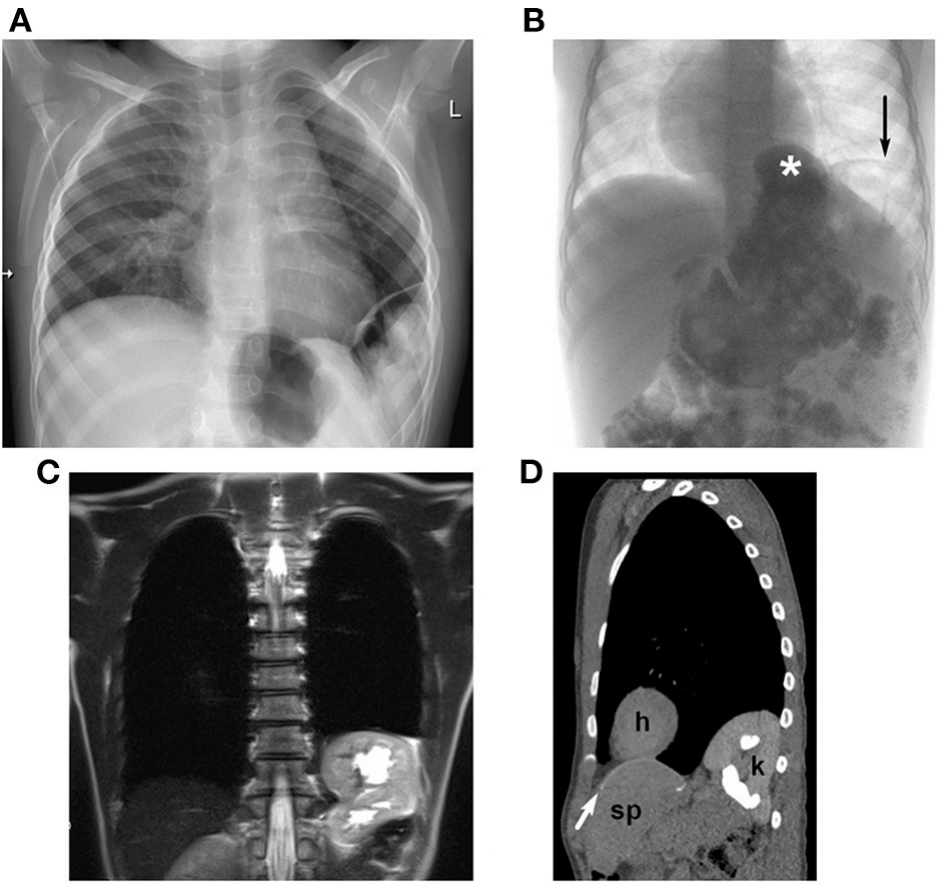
Radiological diagnosis of recurrence of diaphragmatic hernia: (A) plain chest-X-ray in a 2-year-old boy: lateral recurrence; (B) contrast study in a 4-year-old boy: hiatal hernia (^*^) and lateral recurrence (→); MRI (C) and low-dose CT (D) in a 10-year-old girl with thoracic herniation of the left kidney with moderate hydronephrosis [CT scan (D): h, heart; k, kidney; sp, spleen; cone-shaped patch marked with white arrow].
